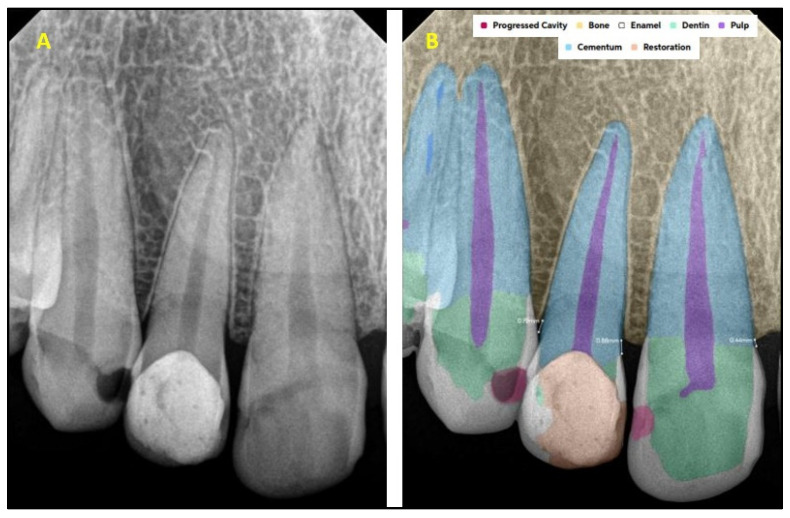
(A) Intraoral periapical radiographic image of maxillary anterior teeth; (B) analysis made by artificial intelligence software depicting diagnosed conditions (dental caries, restoration, bone loss).
